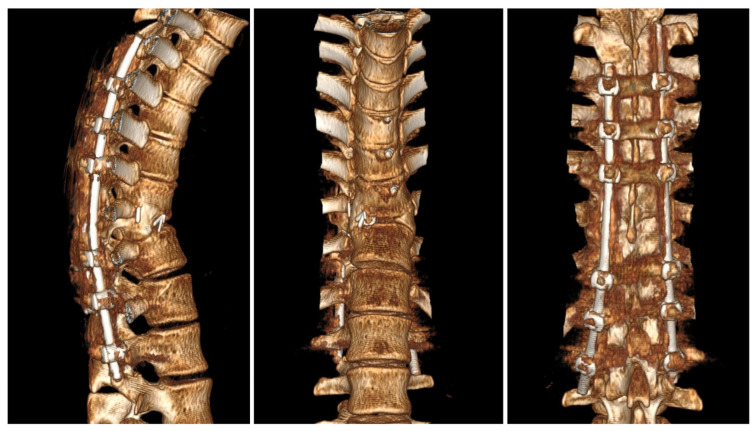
Three-year postoperative CT imaging demonstrating complete posterior spinal fusion of the operated spinal segments, with continuous trabecular bone formation across the fusion site and stable instrumentation. No signs of pseudarthrosis, implant failure, or loss of correction were identified.
